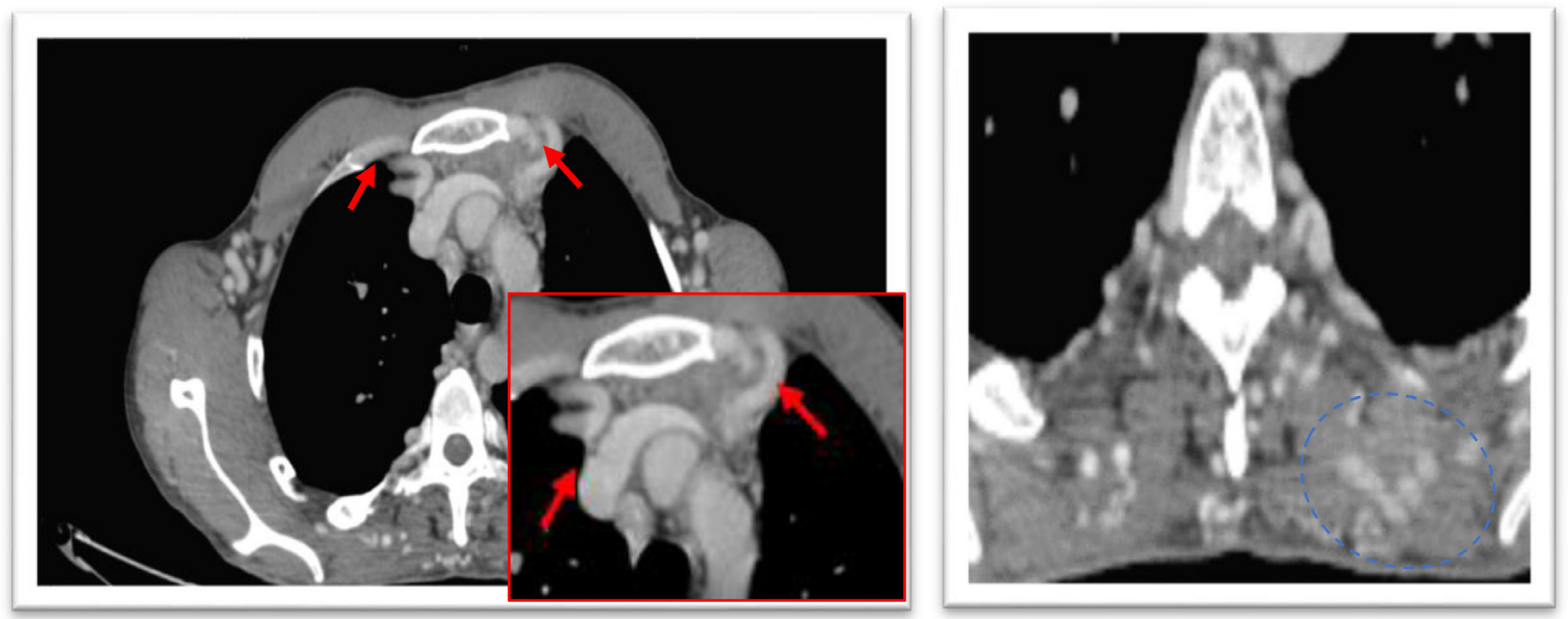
Axial-enhanced thoracic CT scan showing: Extensive collateral arterial circulation involving the intercostal, internal mammary (red arrow), bronchial, epigastric, and posterior chest wall (blue circle) muscle plane arteries which are dilated. Provides a bypass route for the poststenotic descending aorta with recovery of a satisfactory caliber downstream measured at 21 mm poststenotic and 13 mm (abdominal suprarenal aorta).
